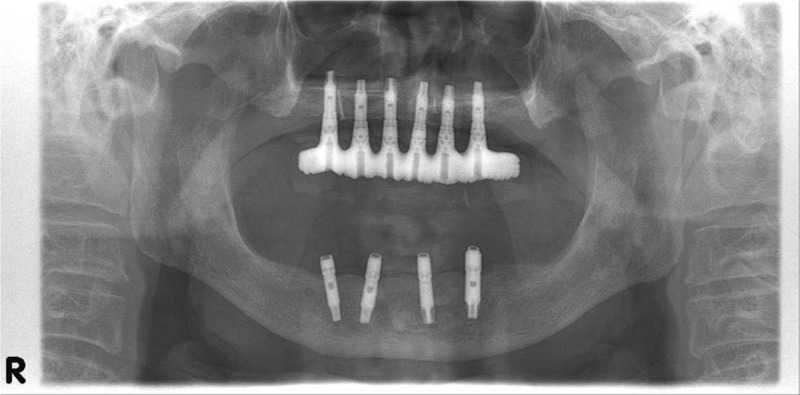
Panoramic radiograph after 11 years of follow-up (March 2019).
